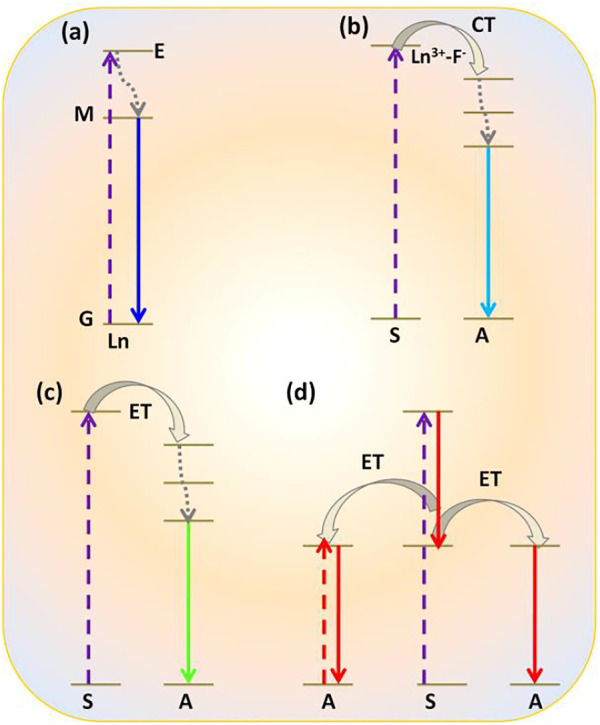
Photophysical processes shown by RE^3+^-doped nanomaterials: (A) downshifting; (B) charge transfer (CT); (C) energy transfer (ET); (D) quantum cutting downconversion.
